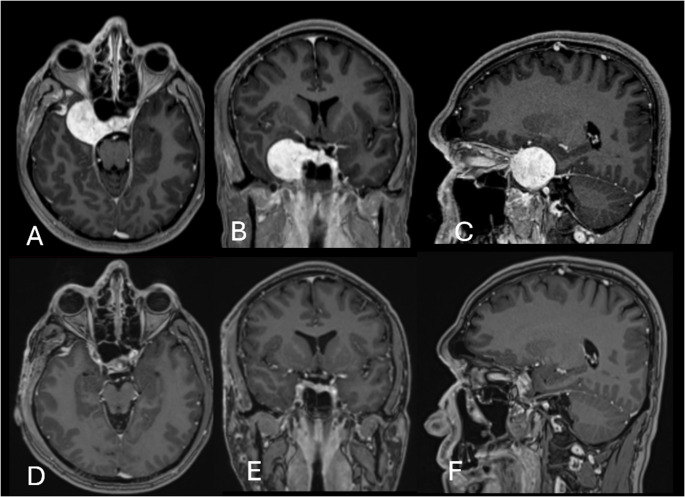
Imaging studies of Case 2 (Table 1), a 33-year-old male with a right CSH managed with HfGKRS. Axial (A), coronal (B), and sagittal (C) T1-weighted contrast-enhanced MR images obtained on the day of treatment, and corresponding images (D, E, and F) acquired 4 years later demonstrate complete volume reduction
